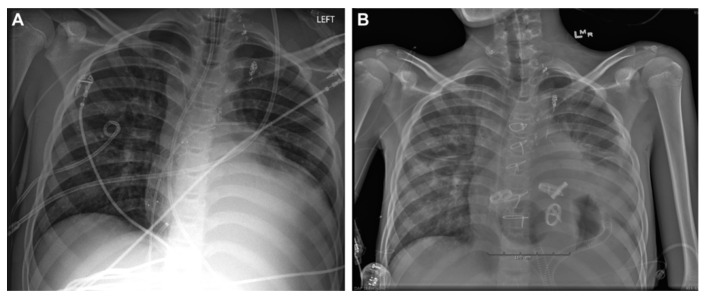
Patient with Fontan circulation implanted with SynCardia™. Image courtesy of Rossano et al. ATS 2014. (A) The preoperative and (B) postoperative chest x-rays. Note the four artificial valves.
